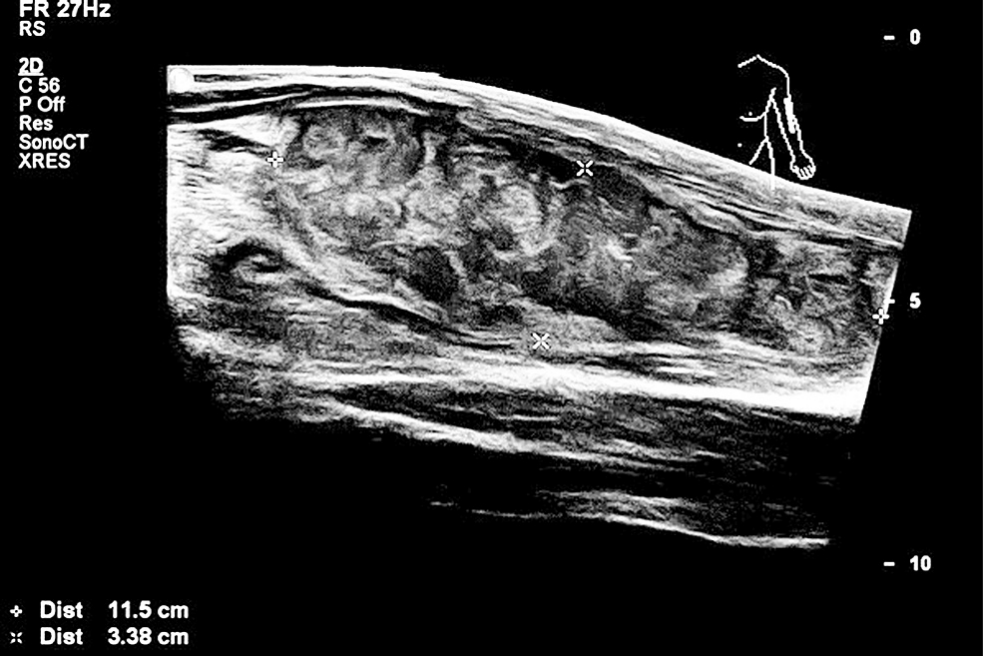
Ultrasonography of the elbow showing a hematoma
